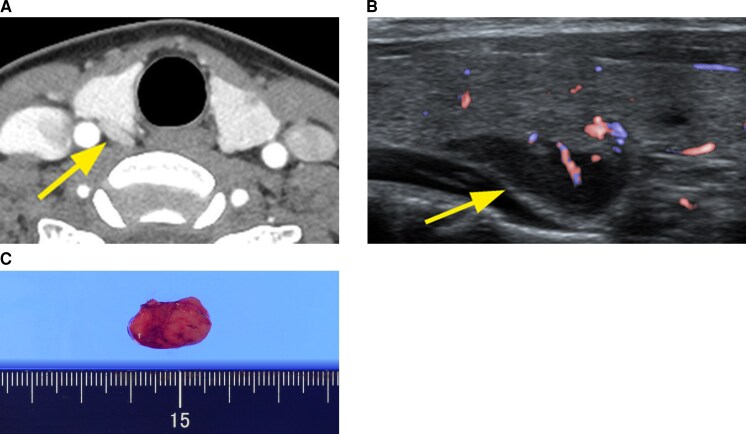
Imaging and macroscopic findings of the 550-mg parathyroid lesion. (A) Four-dimensional computed tomography image showing a corresponding lesion (arrow). (B) Ultrasonography image demonstrating a well-defined hypoechoic lesion posterior to the lower pole of the right thyroid lobe (arrow) with internal vascularity. (C) Gross resected parathyroid adenoma (550 mg).
